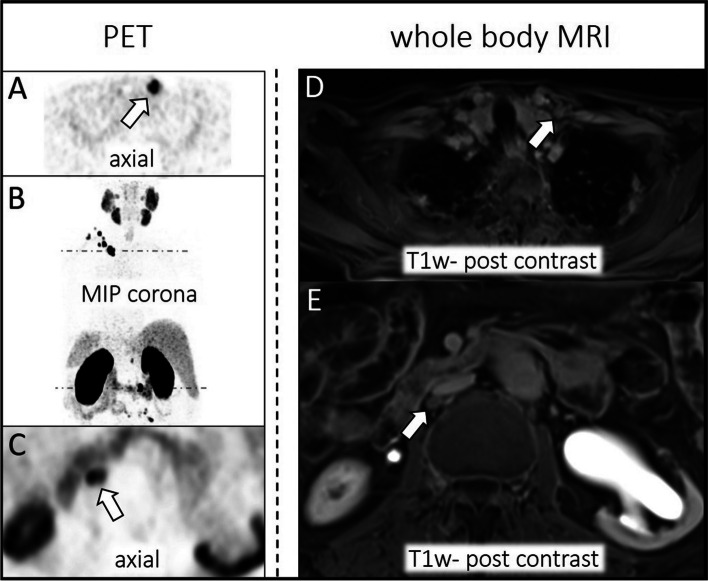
Distant lymph node recurrence of PCa. Cervical (A) and retroperitoneal (C) lymph node metastases detectable by strong tracer accumulation (SUVmax: 60.3) at ^68^Ga-PSMA-11 PET (left A-C, white arrow: MIP corona, axial datasets). Dashed line in MIP corona (B) indicates axial PET layer (A, B). No pathological rating cervical (D) and retroperitoneal (E) at mpMRI (right, white arrow: T1w post contrast)
